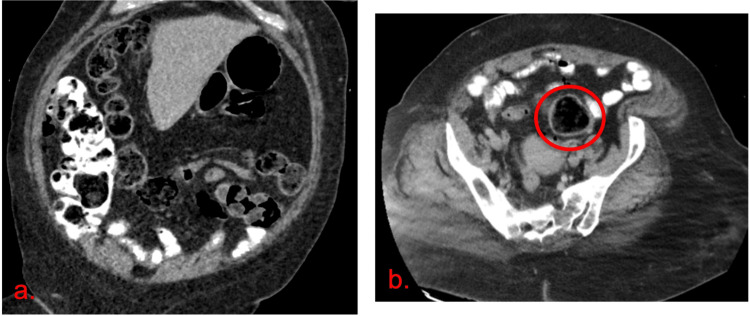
CT abdomen/pelvis. (a) Stool throughout the colon with contrast penetrating through the entire length of colon. (b) Sigmoid colon (red circle) without signs of colitis or fecal impaction.
